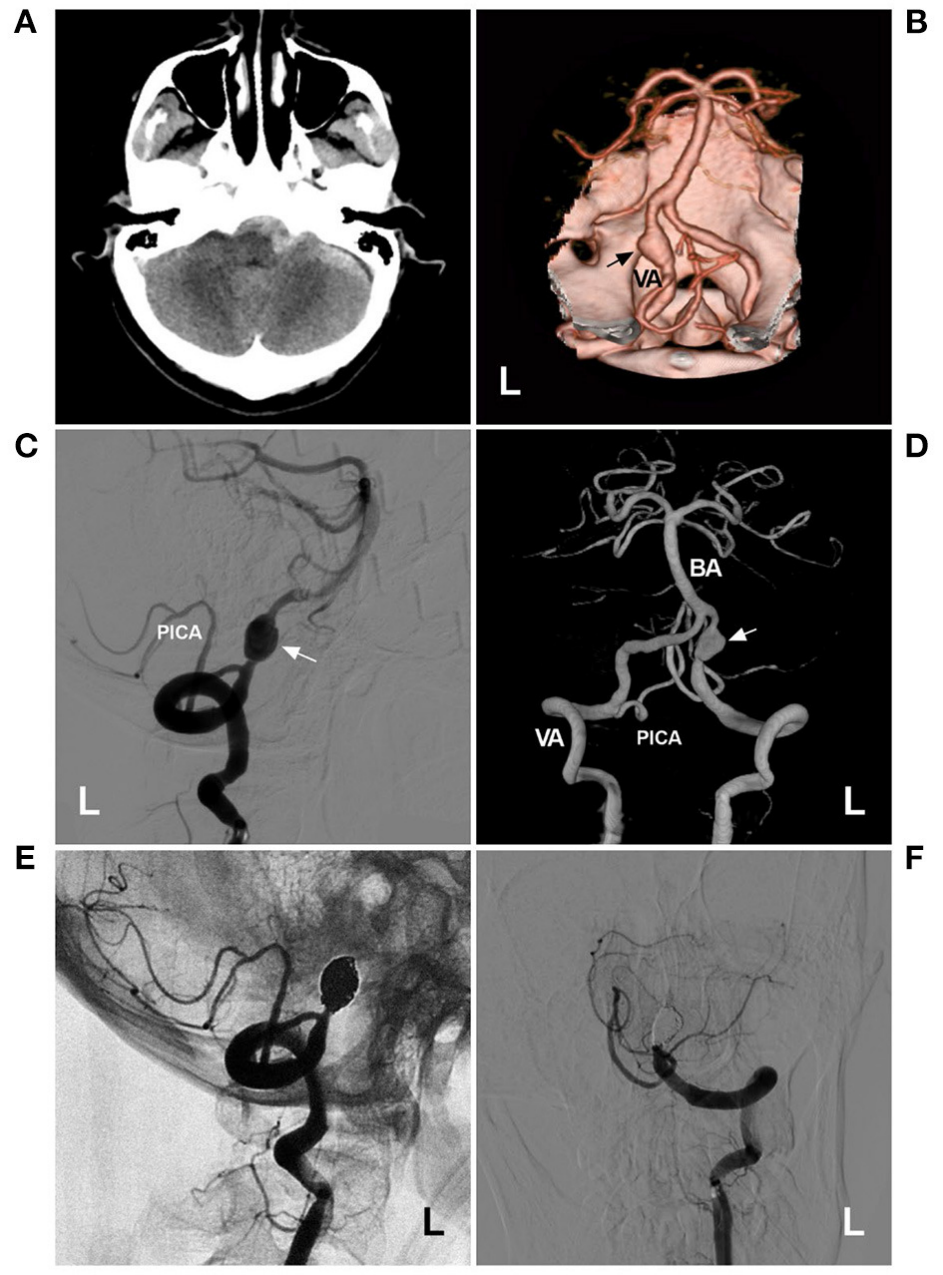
PAO for a VA acute fusiform aneurysm. (A) CT showed subarachnoid hemorrhage in front of the brainstem. (B) CTA showed a fusiform aneurysm (arrow) on the left VA. (C) DSA of the left VA showed the aneurysm (arrow) above PICA origin. (D) Three-dimensional DSA showed the aneurysm (arrow), and the contralateral VA was normally developed. (E) Unsubtracted DSA showed that the aneurysm was treated with PAO, and the PAO was intact. (F) Half-year follow-up DSA showed no recurrence of the aneurysm.BA, basilar artery; CT, computed tomography; CTA, computed tomography angiography; DSA, digital subtraction angiography; L, left; PICA, posterior inferior cerebellar artery; VA, vertebral artery.
